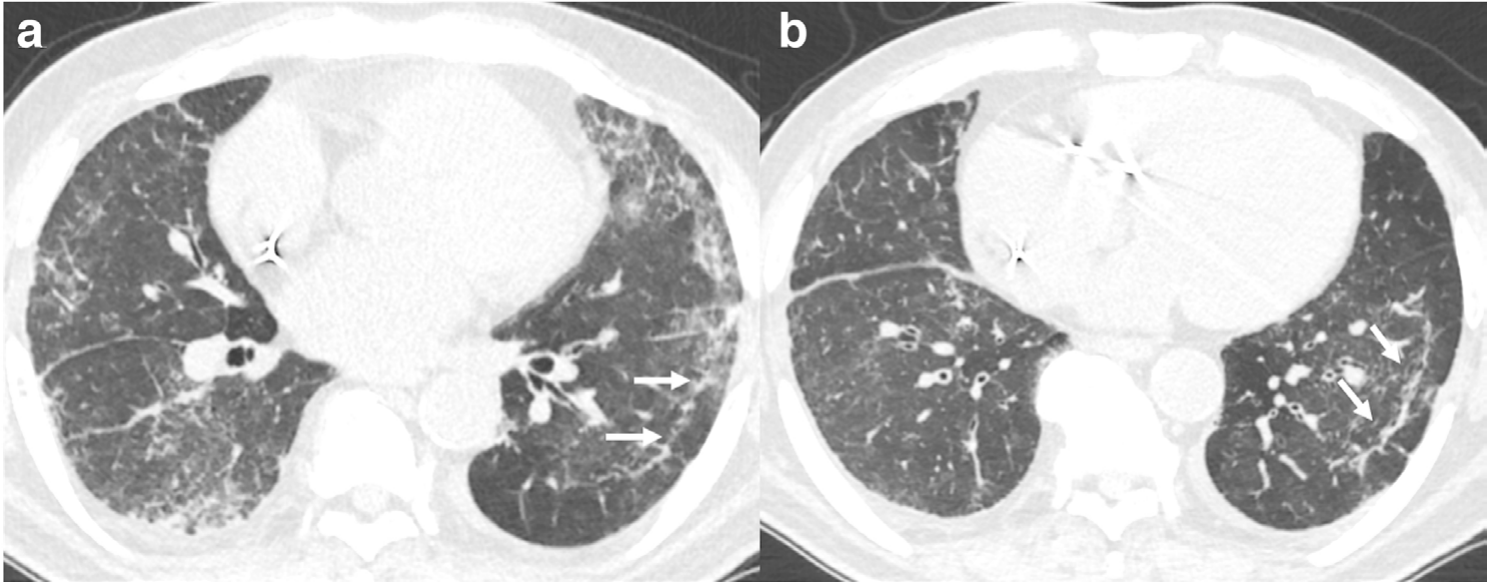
Axial CT chest images (A, B) in a patient with resolving COVID-19 infection. Images show predominantly peripheral bilateral disease with developing subpleural lines in subpleural left lung (white arrows).
